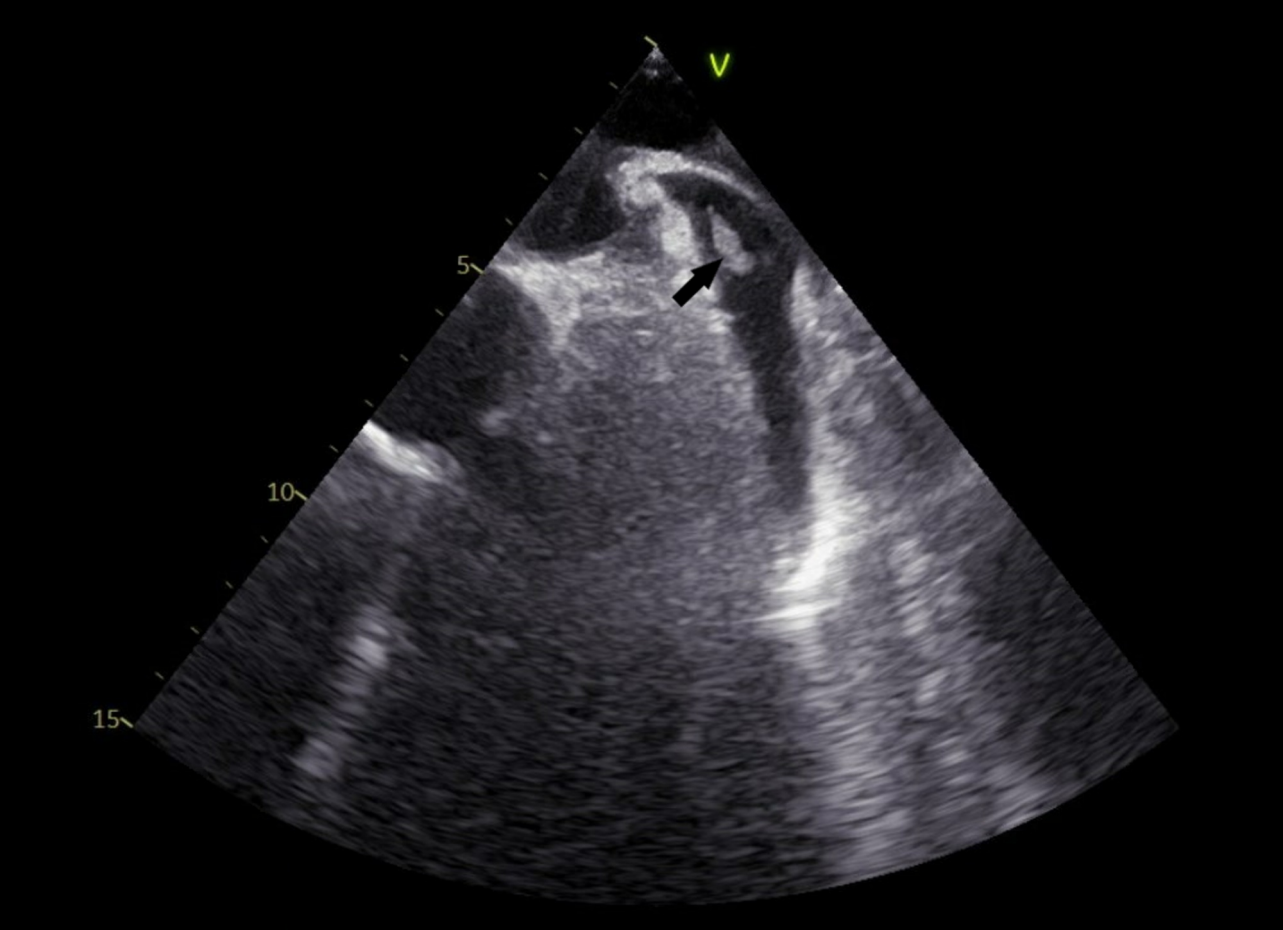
Transesophageal echocardiogram Left atrial appendage thrombus can be seen (arrow).
